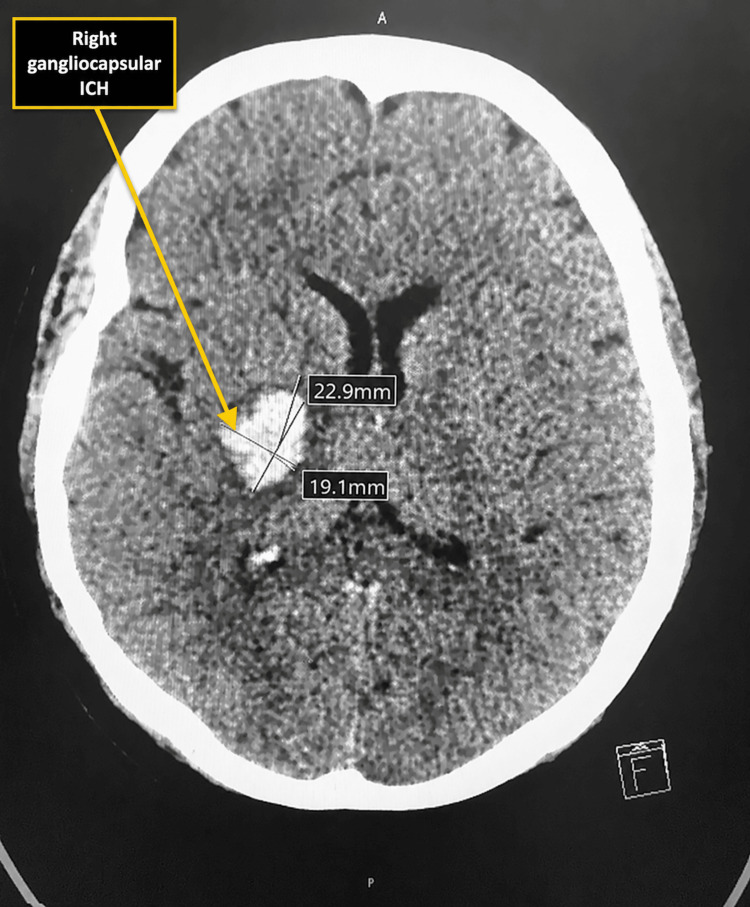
Axial non-contrast computed tomography A hyperdense intraparenchymal hemorrhage is present in the right gangliocapsular region (measuring approximately 22.9 by 19.1 mm in plane), with a smaller concurrent right-sided focus. The deep location is compatible with hemorrhage related to moyamoya collateral rupture but also overlaps with common hypertensive intracerebral hemorrhage locations; given the patient's hypertension, it is not specific and is interpreted together with the computed tomography angiography findings.
